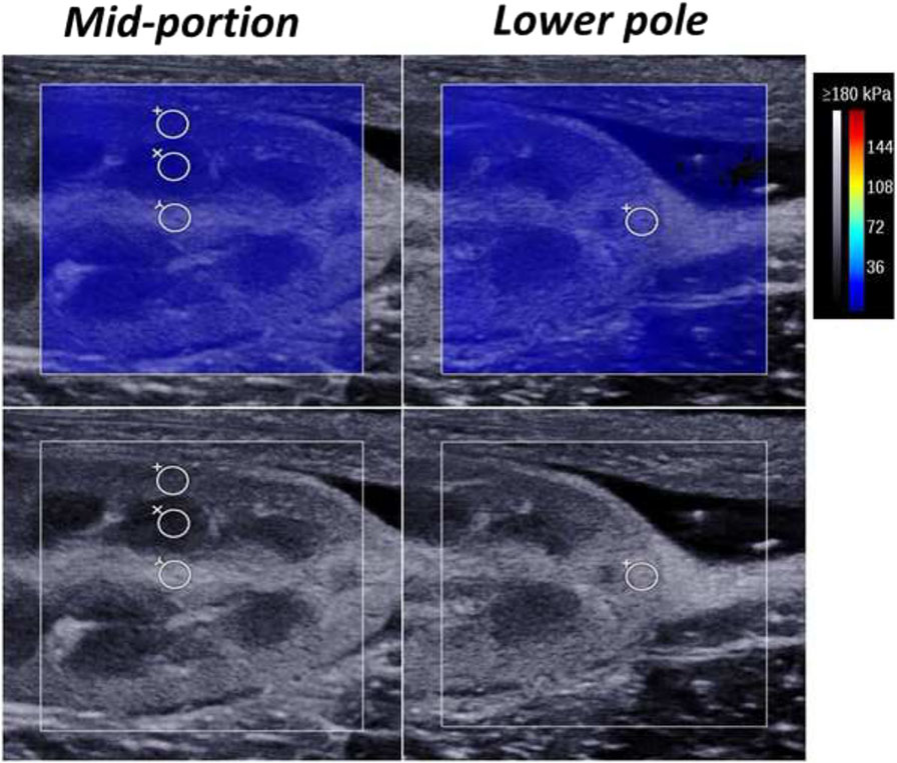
Representative images of elasticity measurement by shear wave elastography (SWE) in one kidney. The regions of interest (ROIs) were positioned in the cortex, medulla and sinus of the mid-portion and in the cortex of the lower pole using the B-mode image. The lower and upper rows show the changes in the ROI by grey-scale ultrasound and SWE, respectively. The colour map is the distribution of elasticity values scaled from 0 to 180 kPa and calculated from shear wave velocity (SWV) values using Young’s modulus (YM)
